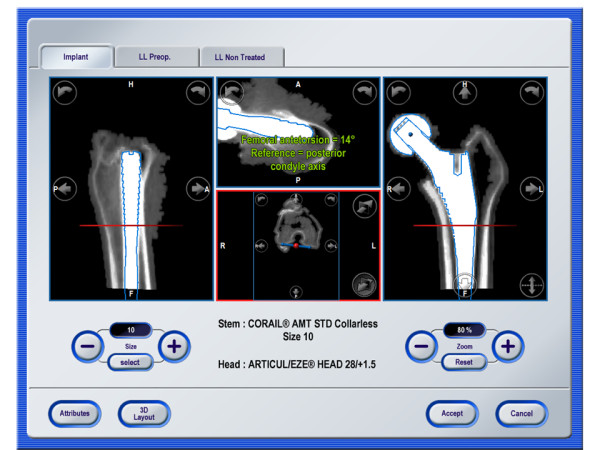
Alignment of stem implant and planning of landmarks. The geometric CAD model of the implant is superimposed onto the CT data set and antetorsion is measured as the angle between the posterior condyle axis landmarks and the implant neck axes in an axial projection.
